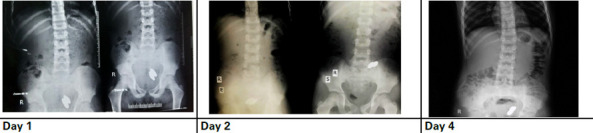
Serial radiographs done to check for progression of foreign body.
